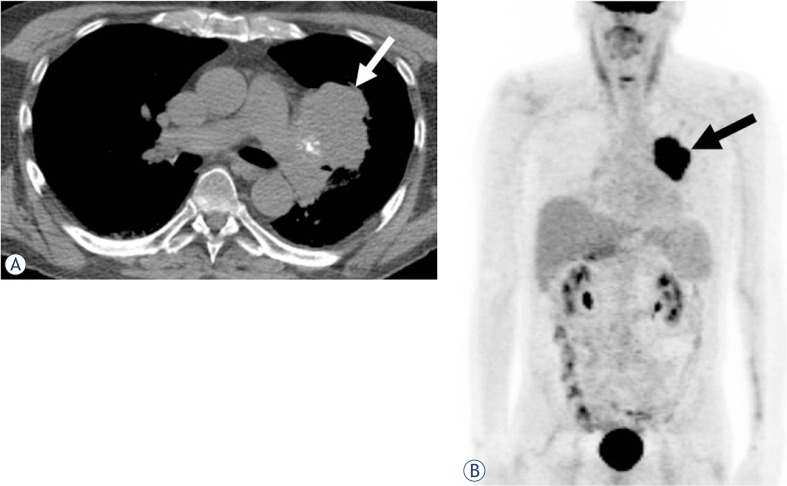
A 67-year-old man with MPNST involving the left lung. A. Unenhanced CT image of the chest at the level of main pulmonary arteries shows a lobulated mass abutting the left hilum with peripheral foci of calcification (arrow). B. Coronal maximum intensity projection (MIP) image from PET/CT shows an intensely fluorodeoxyglucose (FDG) avid mass (arrow, standardized uptake value [SUV]_max_ 23.6). Patient underwent left pneumonectomy on which intrapulmonary location of the mass was confirmed. Rhabdomyoblastic differentiation (malignant triton tumor) was noted on pathology.
